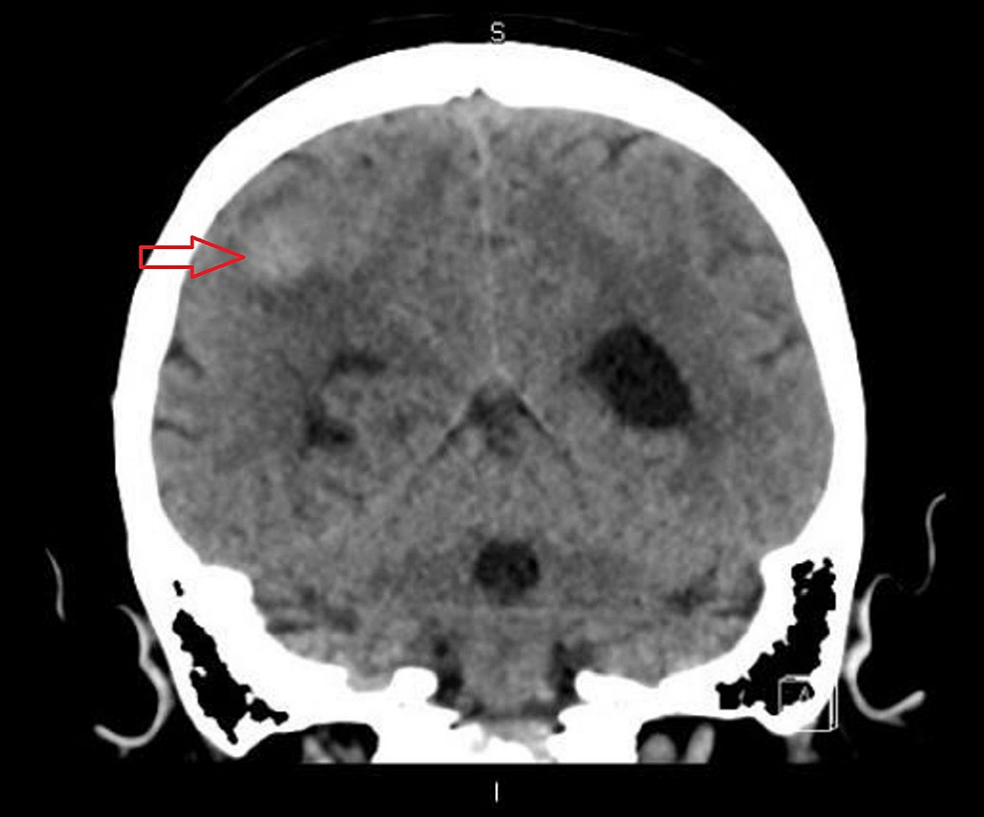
CT Head (Coronal View) Showing Hyperdense Lesion in the Right Posterior Parietal Lobe
